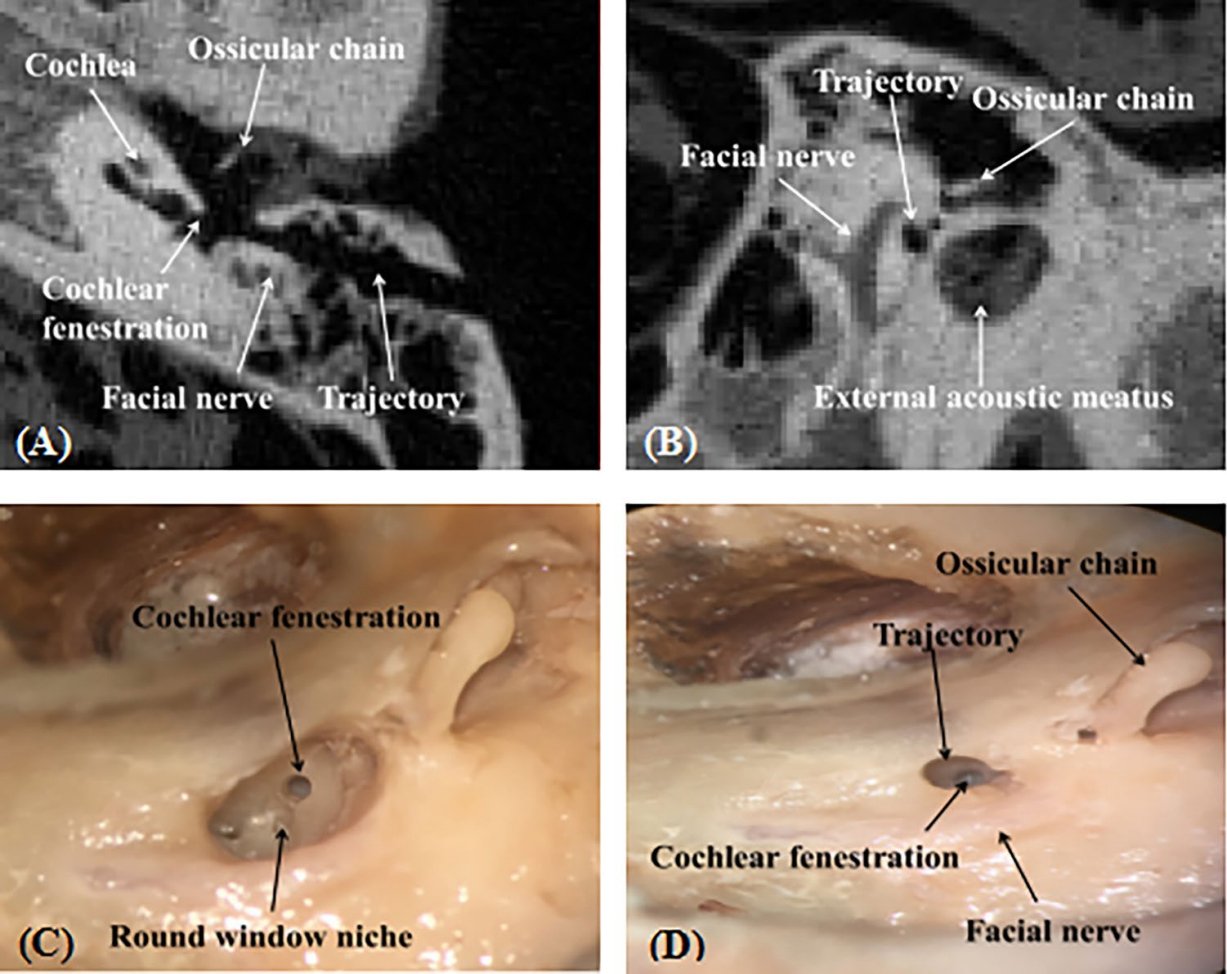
(A, B) The location between the trajectory and surrounding anatomic structures in high-resolution computed tomography. (C) The location relationship between the drill path and facial nerve in routine temporal anatomy. (D) The target point is on the basal turn of the cochlea.
